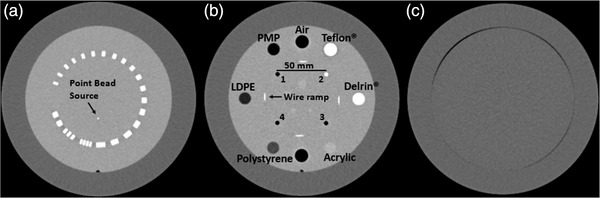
Computed tomography (CT)‐scans of the CatPhan sections CTP528 (a; used for MTF determination), CTP404 (b; contains inserts, rods and ramps for determining CT number accuracy, contrast‐noise‐ratio (CNR), spatial linearity, and reconstructed slice thickness) and CTP486 (c; used for evaluating uniformity and noise characteristics). The rods in CTP404 are labeled for distinctiveness with the numbers 1– 4. The non‐labeled insert at 6 o'clock position in (b) represents an additional, second air insert
